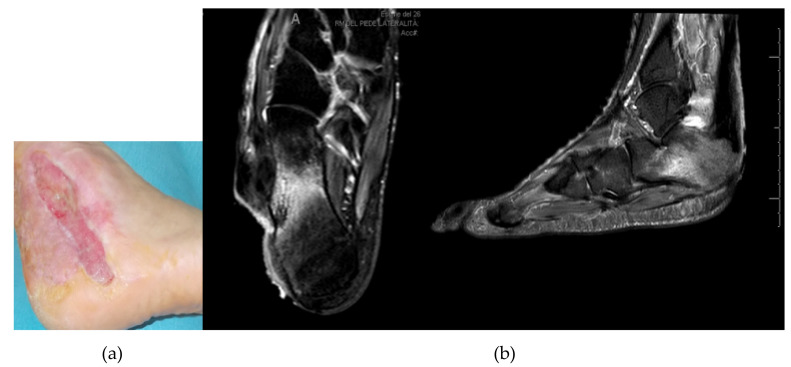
Photograph (a) and MRI (b) showing infection after insufficient first surgical approach with resection of the lesion and dermal substitute application.
